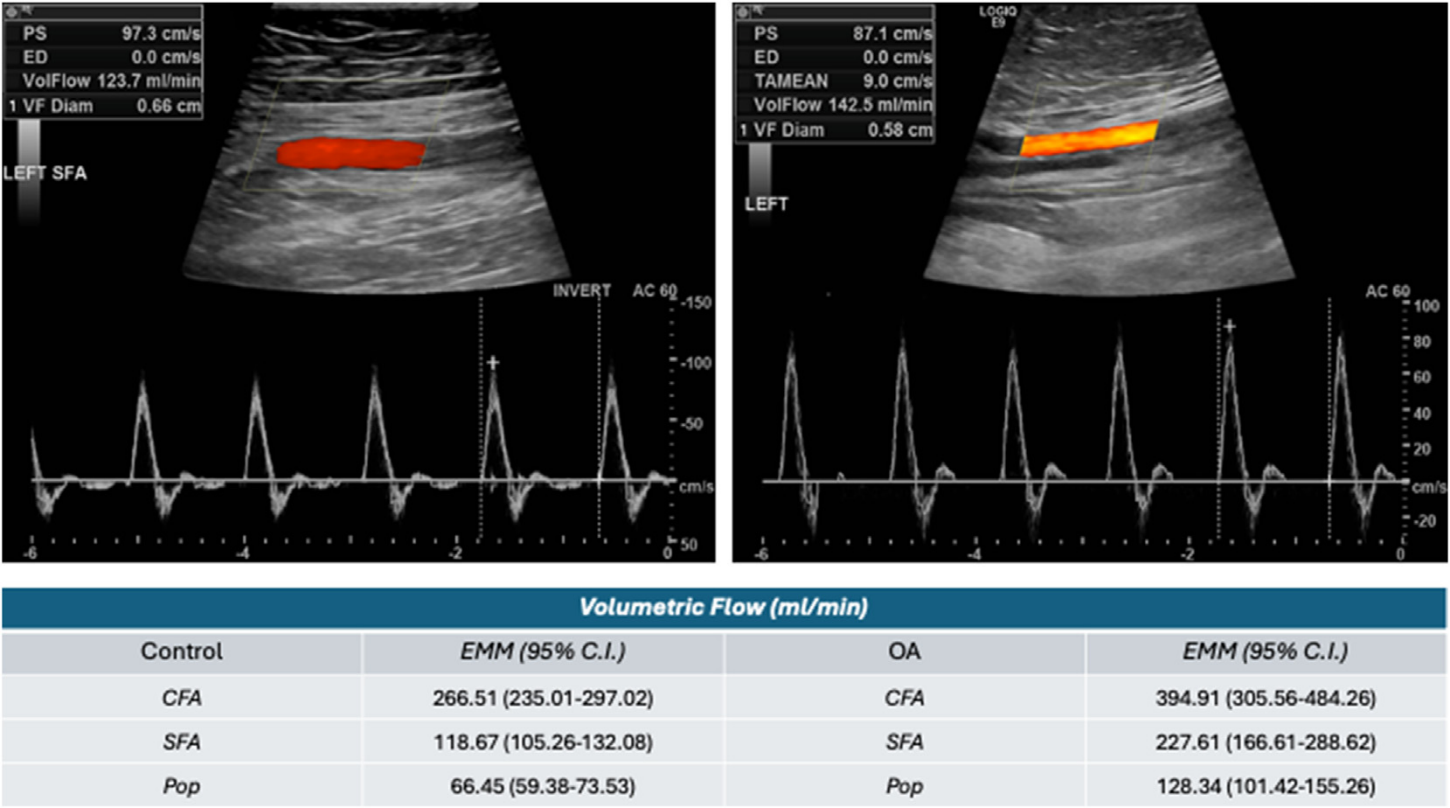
Limb arterial volumetric flow sonographic imaging captured using the Logiq9 system. Laminar flow was observed in all major vessels studied. Volumetric flow was significantly greater in all three vessels of OA knees than in the vessels of control knees (CFA *P* ​< ​0.001; SFA *P* ​< ​0.001; Pop *P* ​< ​0.001). EMM ​= ​estimated marginal mean.
